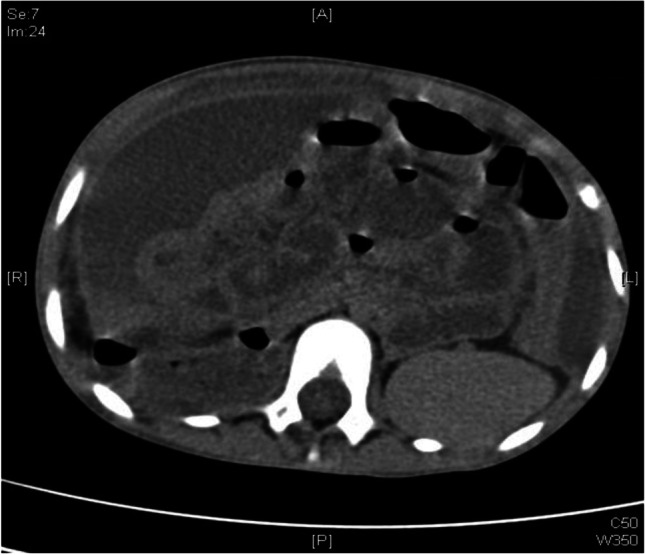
Pre-operative CT-scan of *Case 2* showing profuse turbid ascites, thickening of the intestinal wall, intestine tethered to the spinal column, but no peritoneal calcifications
